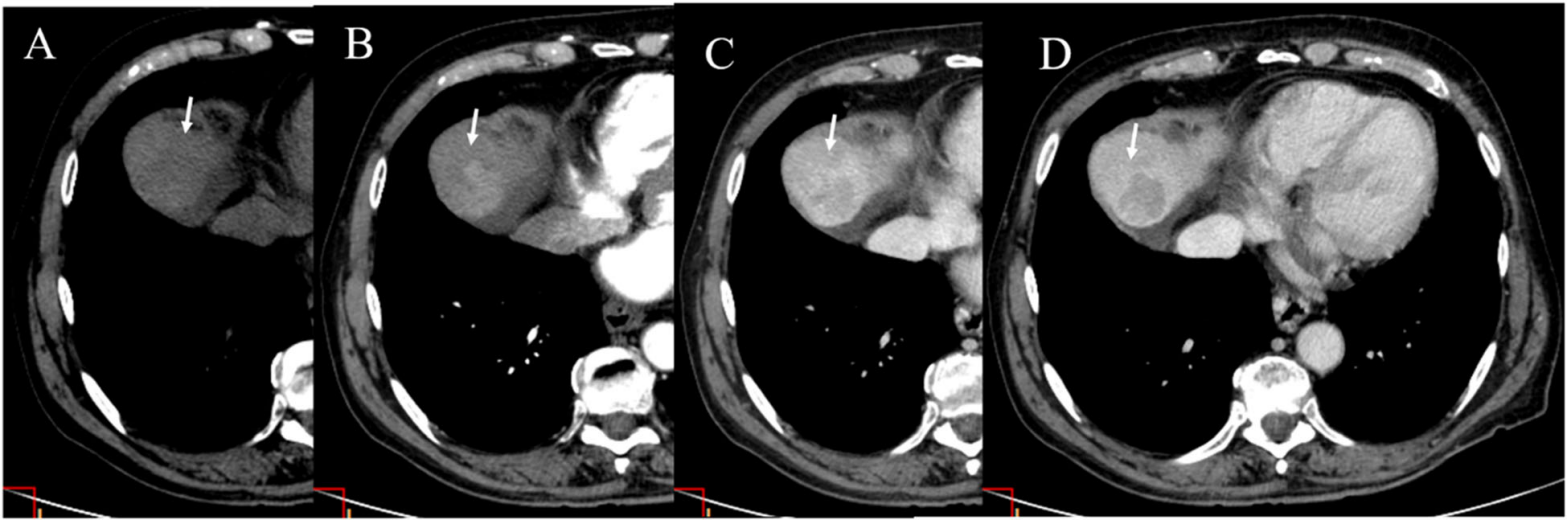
CT assessment of HCC on IVa hepatic segment. In (A) pre contrast phase. During contrast study, the lesion (arrow) shows hyperenhancement during artierial phase (B), wash out during portal (C) and capsule appearance in equilibrium phase (D)
